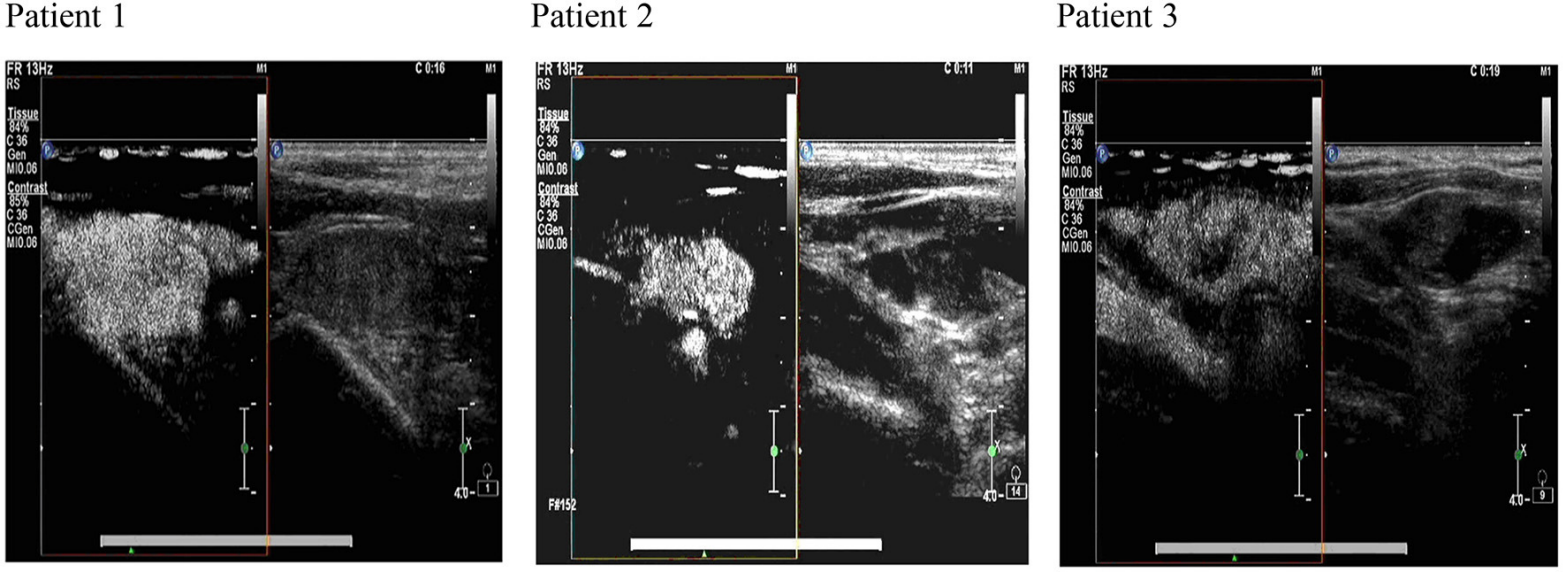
The ultrasonography image of cervical lymph nodes in comparison with ultrasound contrast and color ultrasound images. The images produced by color ultrasound are used for the evaluation of the nature of lymph node lesions. The characteristics of “uneven enhancement” and “fast forward and slow backward” are observed after ultrasound contrast. Postoperative pathological analysis confirms lymph node metastasis.
